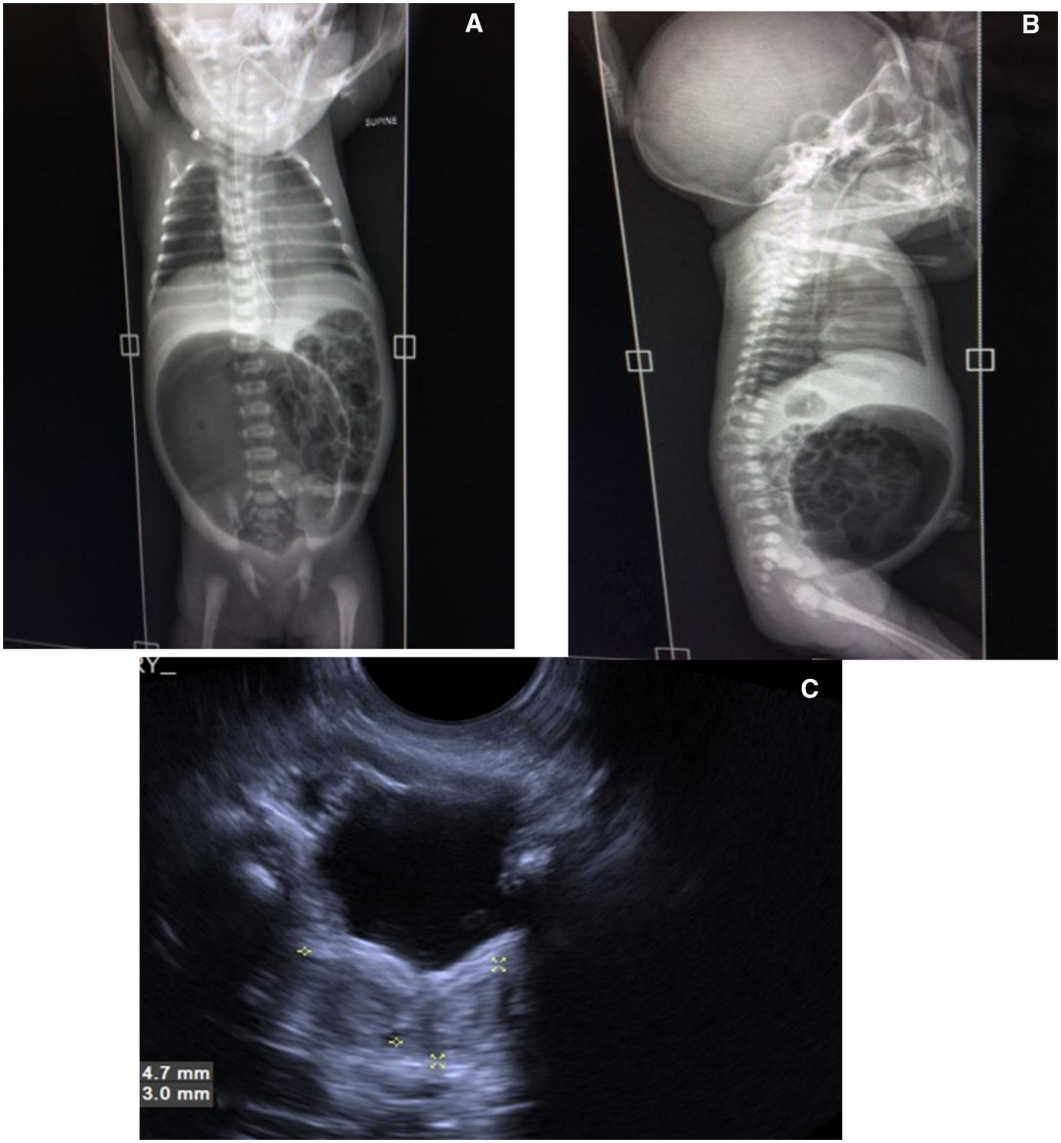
(A–C) Postnatal imaging at 24 h of life. Xray abdomen supine AP (A) and lateral (B) view showing grossly distended air-filled pouch in lower abdomen. USG scan of pelvis of baby (C) showing Didelphis uterus (marked by asterisks).
